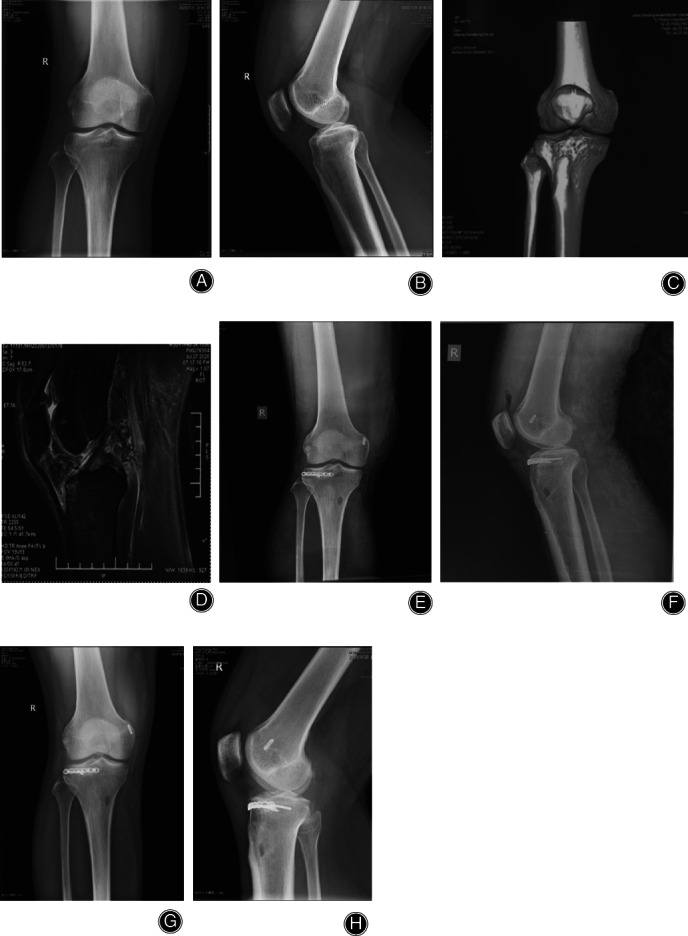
Hyperextension bicondylar fracture. Male, 42 years old. (A, B) No fracture can be seen on X‐Ray. (C) 3D CT showed the compression of the anterolateral tibial plateau. (D) MRI showed the posterior cruciate ligament (PCL) injury. (E, F) The anterolateral articular surface was reduced and fixed with a horizontally‐oriented rim plate; arthroscopic reconstruction of the PCL was performed using the single‐bundle technique. (G, H) The fracture healed and satisfactory reduction had been achieved 1 year postoperatively.
